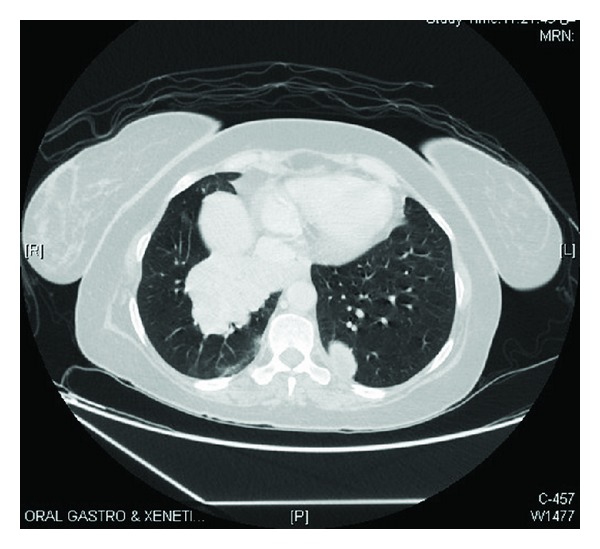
Computed tomography of chest showing largest pulmonary metastatic nodule of size 10 × 6 cm in right middle and lower lobes invading adjacent mediastinum, mediastinal pleura, pericardium, and right pulmonary vein and bronchus.
